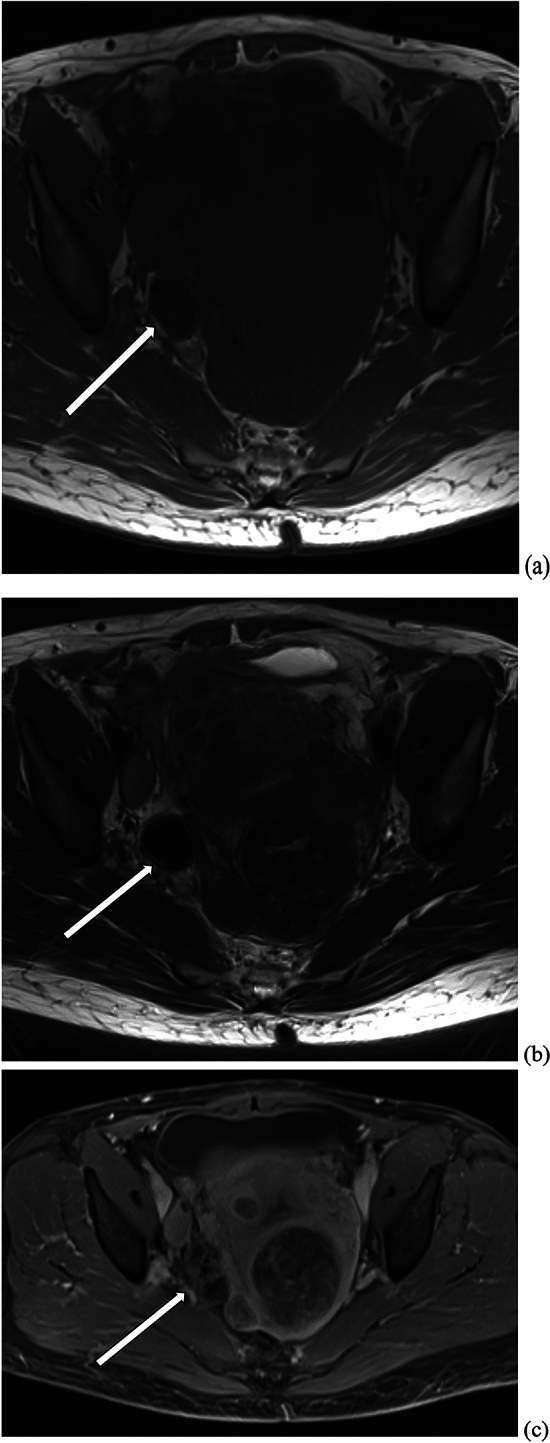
A 68-year-old woman with a past medical history of hypertension and uterine fibroids presented with a 3-year history of hirsutism, with increased hair on shoulders. Laboratory results showed elevated testosterone, suppressed luteinizing hormone (LH) and follicle-stimulating hormone (FSH), and low DHEAS levels. Pelvic MRI showed a right ovarian lesion (arrow) with low to intermediate T1 signal intensity (image a), low T2 signal intensity (image b), and heterogeneous enhancement (image c). On surgical resection, a 2.5 cm right ovarian mass was noted, with final pathology consistent with benign Sertoli–Leydig cell tumor. On follow up visits, the patient reported improving hirsutism and had decreasing testosterone levels
